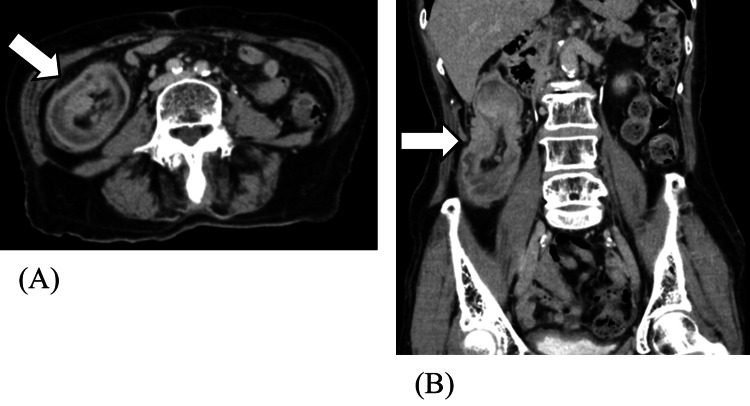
Abdominal contrast-enhanced CT findings at presentation. Axial (A) and coronal (B) slice CT scans of the abdomen show that a 41-mm soft tissue mass with mild enhancement is seen in the cecum (arrow), located at the level of the inferior hepatic margin. A concentric ring appearance (target sign) is visible in the ascending colon, consistent with intussusception, with the tumor as the lead point.
